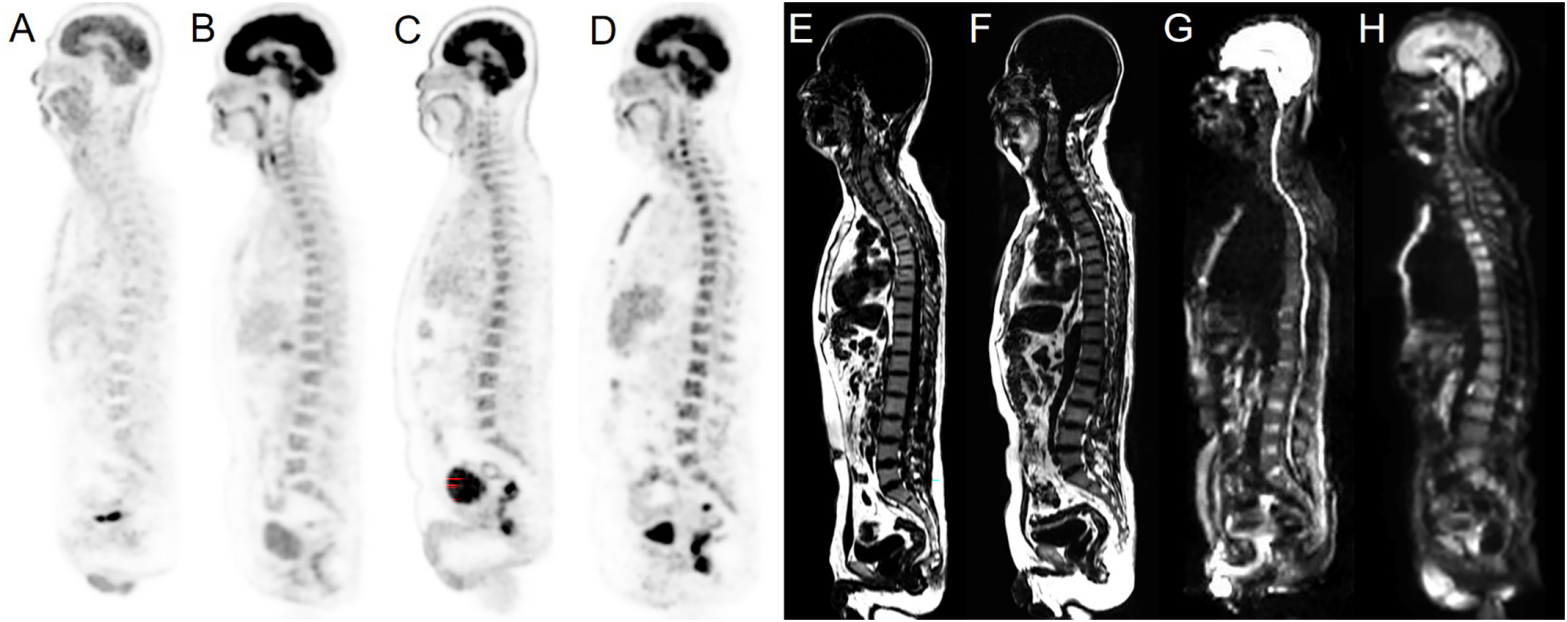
Examples of normal BMs and BMI in our cohort. Sagittal PET images derived from integrated PET/MRI (A–D), T1WI and sagittal DWI images derived from integrated PET/MRI (E–H). Normal (A) and homogeneously increased ^18^F-FDG uptake above the liver parenchyma (B) were considered PET-negative. Heterogeneously increased (C) and unifocal/multifocal FDG uptake above the liver parenchyma uptake (D) were considered PET-positive. Normal signals of BM on the sagittal T1WI image were considered MRI-negative (E). T1WI positivity was defined as decreased signal intensity compared to the surrounding muscles (F). Normal signals of BM on the sagittal DWI image were considered MRI-negative (G). DWI positivity was defined as increased signal intensity compared to the surrounding muscles and intervertebral discs (H).
